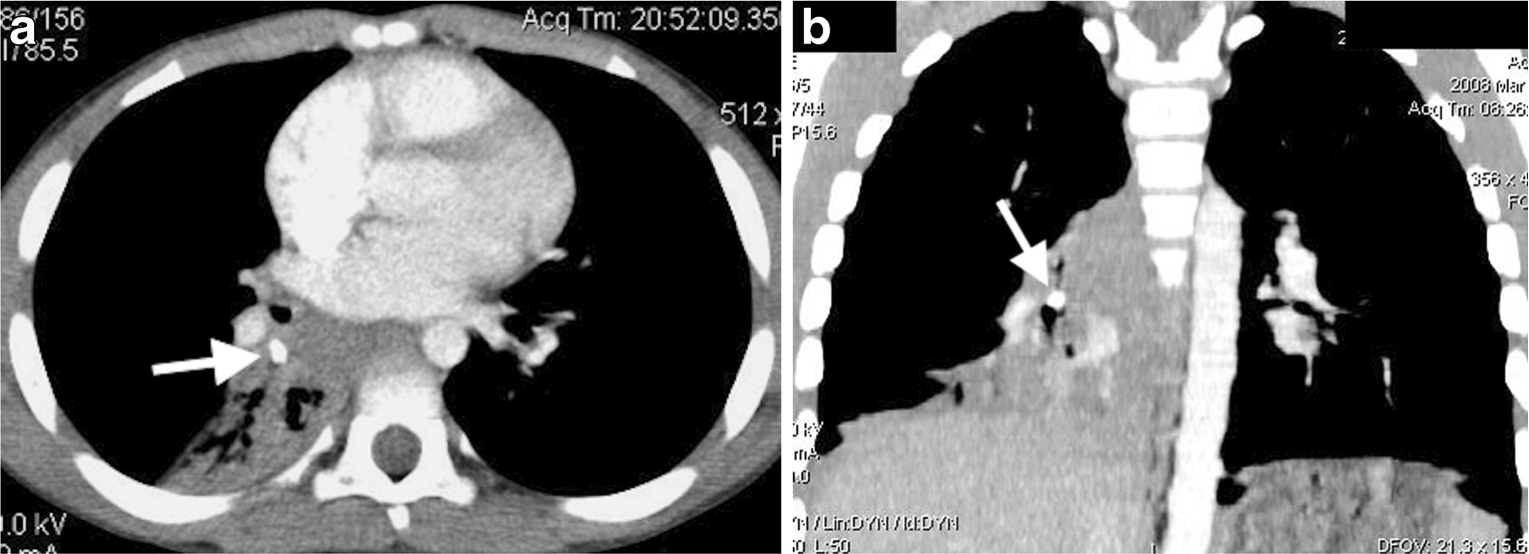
A 9-year old boy with a 6-month history of cough. Contrast-enhanced CT performed for a persistent right lower lobe air-space opacification on chest radiographs demonstrates a dense foreign body (*white arrows*) on axial section (a) and coronal reconstruction (b) as a cause of the right lower lobe posterior segment atelectasis
